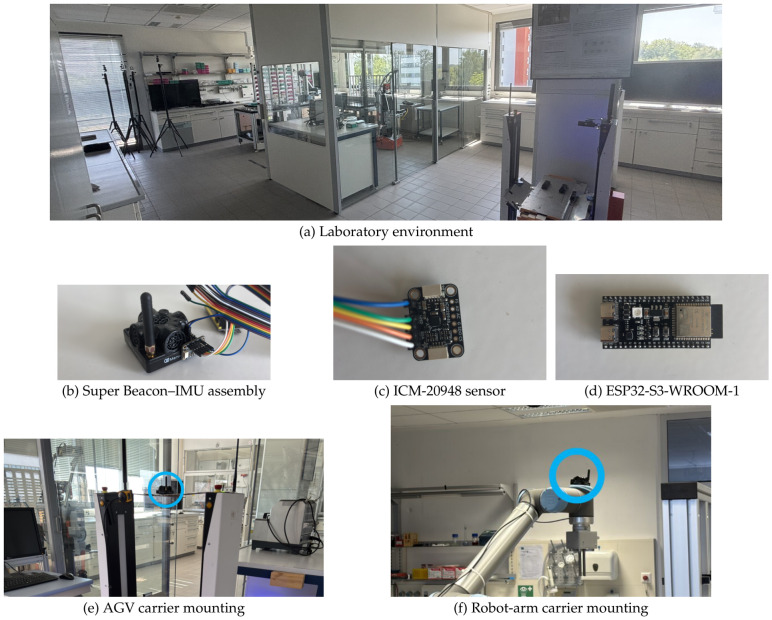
Photographic documentation of the experimental environment, embedded sensing hardware, and carrier mounting configurations. The ultrasonic–inertial sensing node was mounted on the AGV platform for 2D mobile tests and above the UR10 wrist joint for controlled 3D trajectory evaluation; the wrist joint was kept fixed so that the sensor-to-end-effector transform remained constant. The blue circles in (e,f) indicate the mounting positions of the sensors on the AGV and the robotic arm, respectively.
